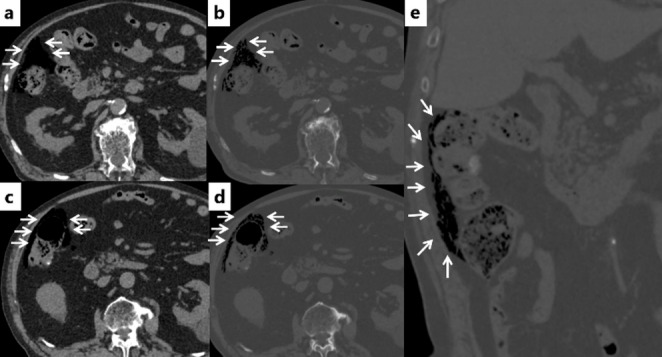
Pneumatosis cystoides intestinalis. a axial CT, b axial CT (bone window), c axial CT, d axial CT (bone window), e coronal CT (bone window). A man in his 80s with type 2 DM presented with no symptoms. He was taking alpha-glucosidase inhibitors to treat DM. Screening CT showed air along the colon wall (a–e; arrow) incidentally, and pneumatosis cystoides intestinalis was suspected. CT reveals multiple cystic collections of gas within the bowel wall without reduced mural enhancement or portal venous gas, suggesting a non-ischemic etiology in the appropriate clinical context (not shown). It disappeared on follow-up CT without any treatment (data not shown). Abbreviations: CT, computed tomography; DM, diabetes mellitus
